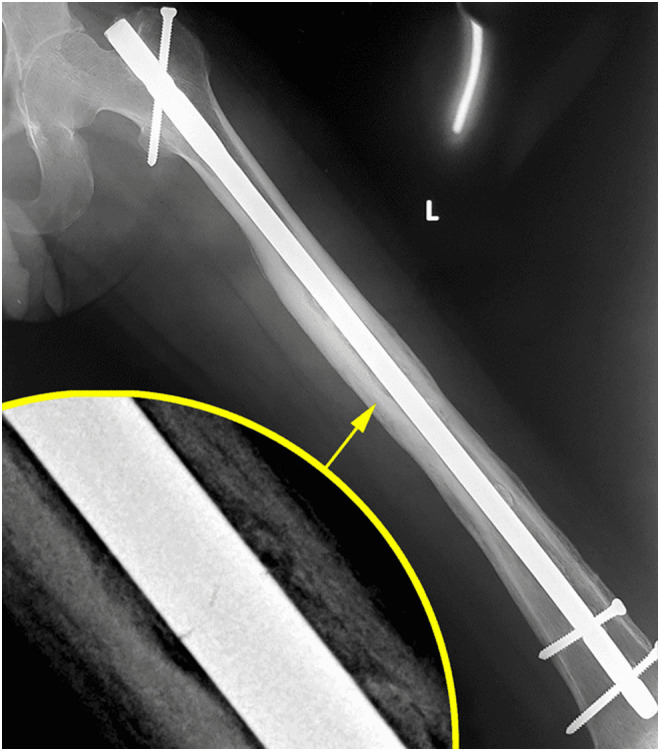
Antero-posterior view of full-length X-ray of the left femur showing broken femur nail with complete bony union.
